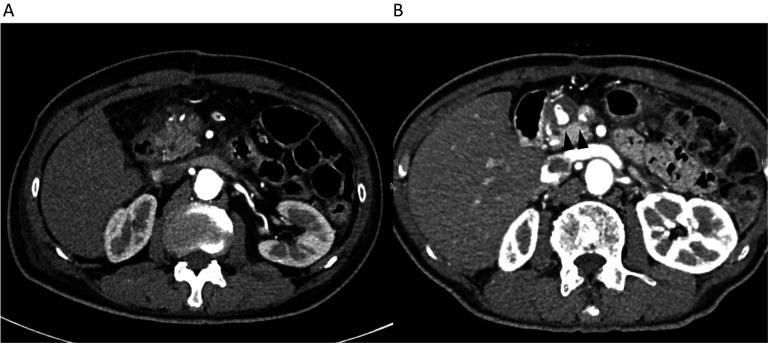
A Contrast-enhanced computed tomography revealed minimal fluid collection and no evidence of pseudoaneurysm on POD7. B Contrast-enhanced computed tomography revealing pseudoaneurysm in the anterior superior pancreaticoduodenal artery (ASPDA) (black arrowhead)
